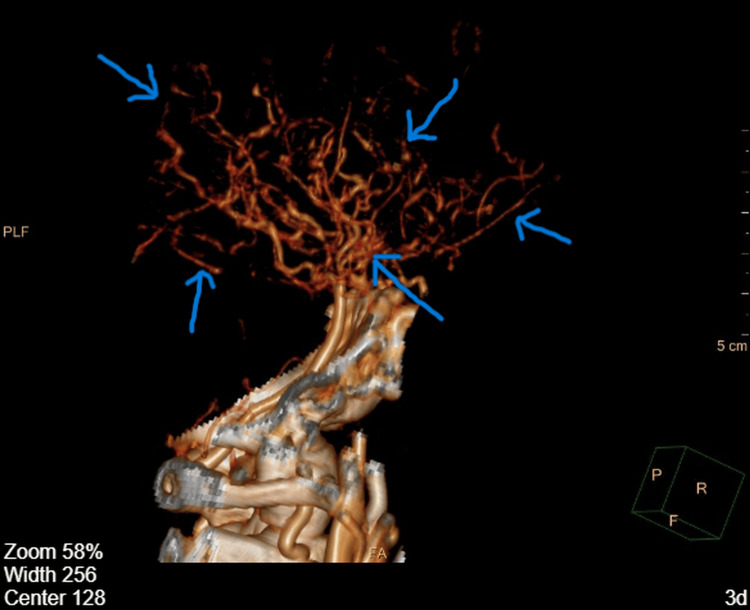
Sagittal three-dimensional CT angiography reconstruction demonstrating collateral vascular network in Moyamoya disease Sagittal three-dimensional CT angiography (CTA) reconstruction of the intracranial vasculature demonstrating abnormal collateral circulation along the lateral aspect of the cerebral hemispheres. These tortuous vascular structures represent compensatory collateral vessels that develop in Moyamoya disease as a response to progressive stenosis or occlusion of the distal internal carotid arteries and their proximal branches. Blue arrows indicate the abnormal collateral vessels forming the Moyamoya collateral network.
